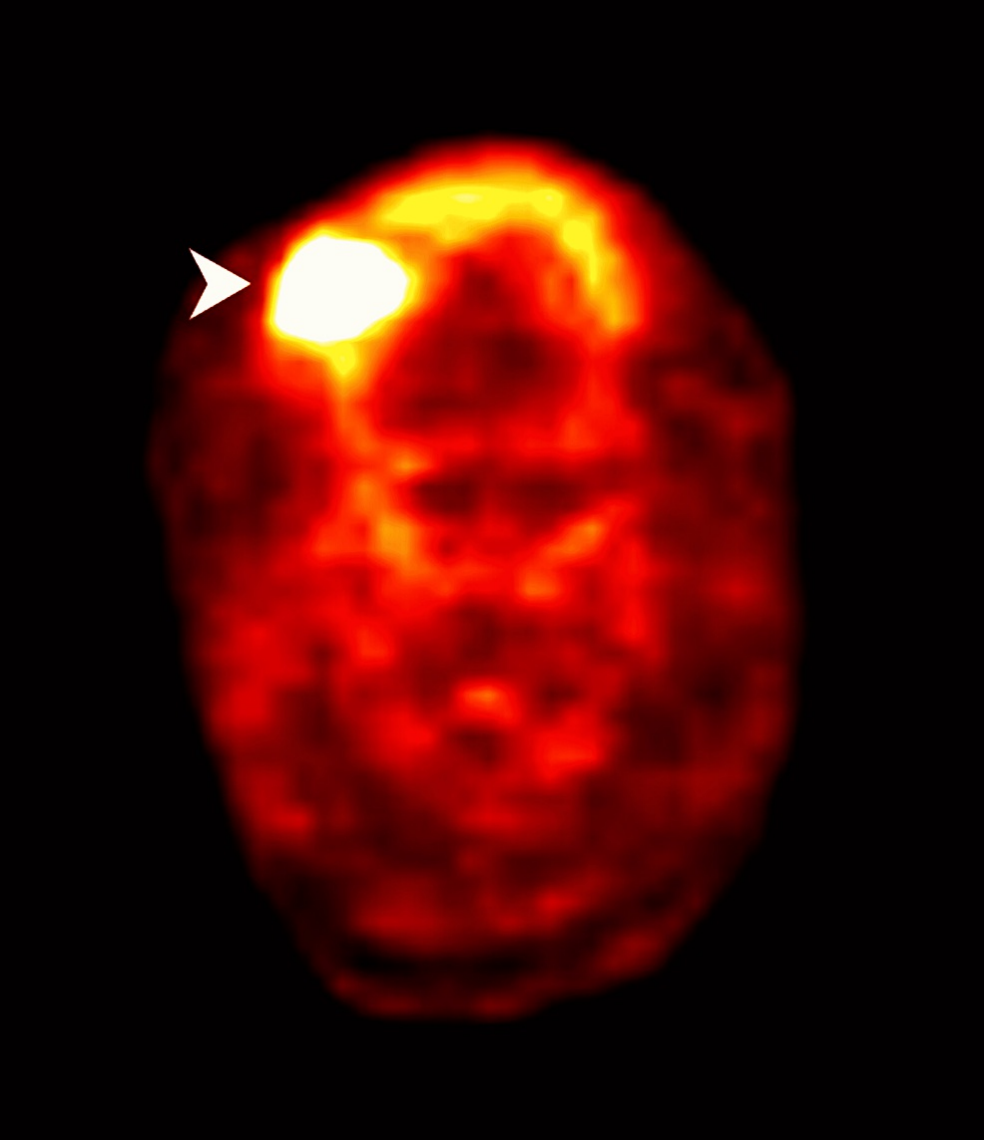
Whole Body PET CT image with PET section of tumour (white arrow head is showing the tumour).
